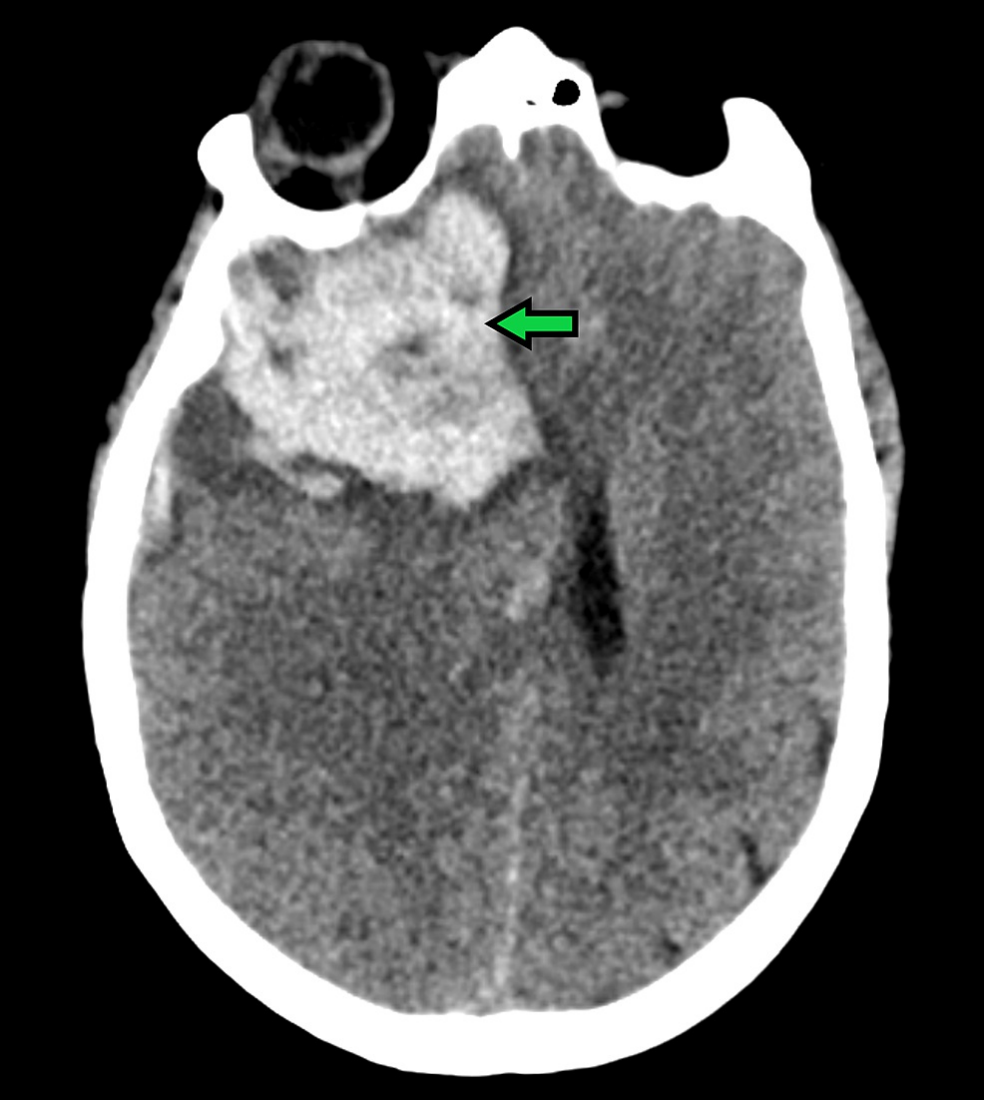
CT of the head showing a large right frontal hematoma with midline shift (arrow) CT: computed tomography
